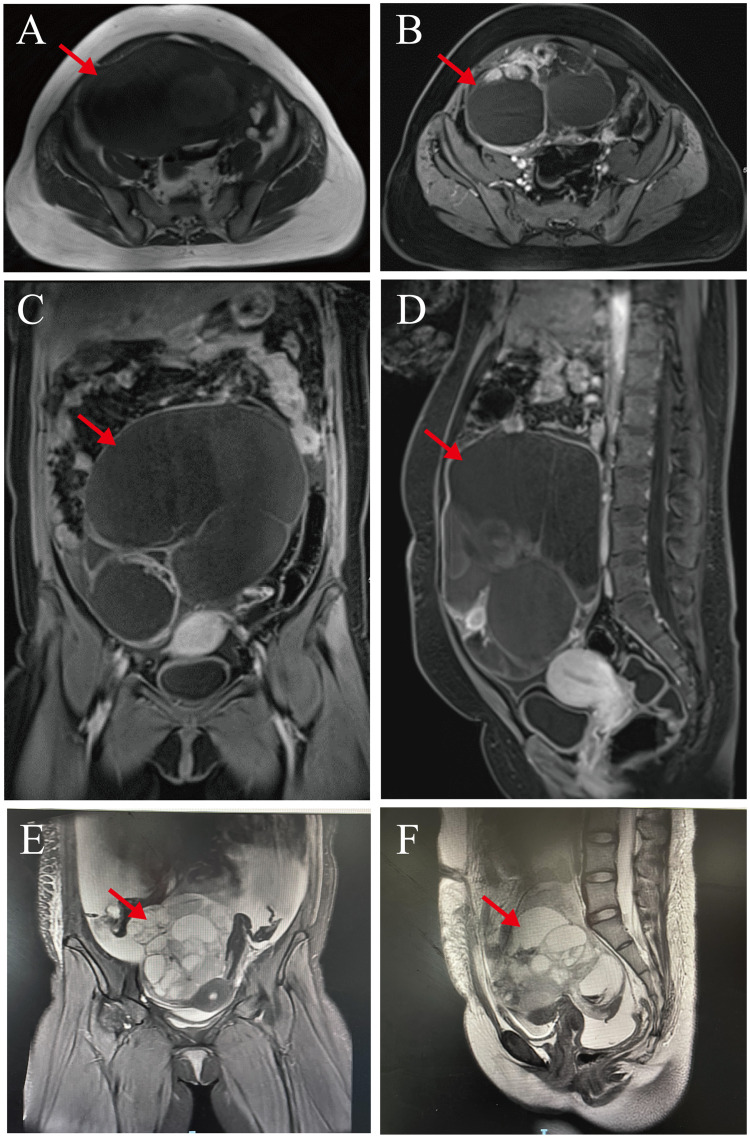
Pelvic magnetic resonance images. (A–D) Preoperative pelvic magnetic resonance images: Abdominopelvic cavity shows a large, cystic solid mass. Solid and septal portions of the lesion show marked inhomogeneity that was in close proximity to the left adnexa (red arrows). (E, F) Three month postoperative pelvic magnetic resonance images: There was a large cystic-solid mass (cross-sectional size of about 122 × 95 × 116 mm) in the pelvis(red arrows), and the adjacent structural tissues were displaced by its compression.
